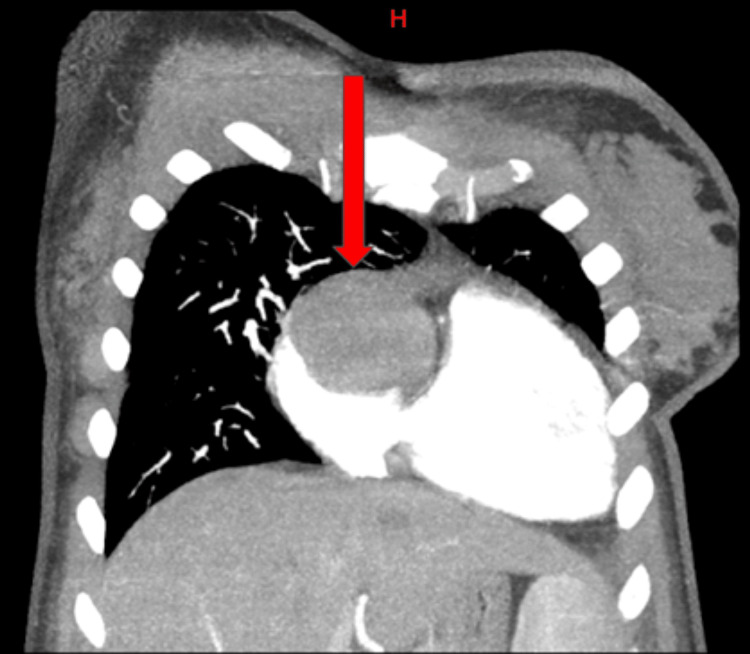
Computed tomography angiography of intracardiac mass H: head
